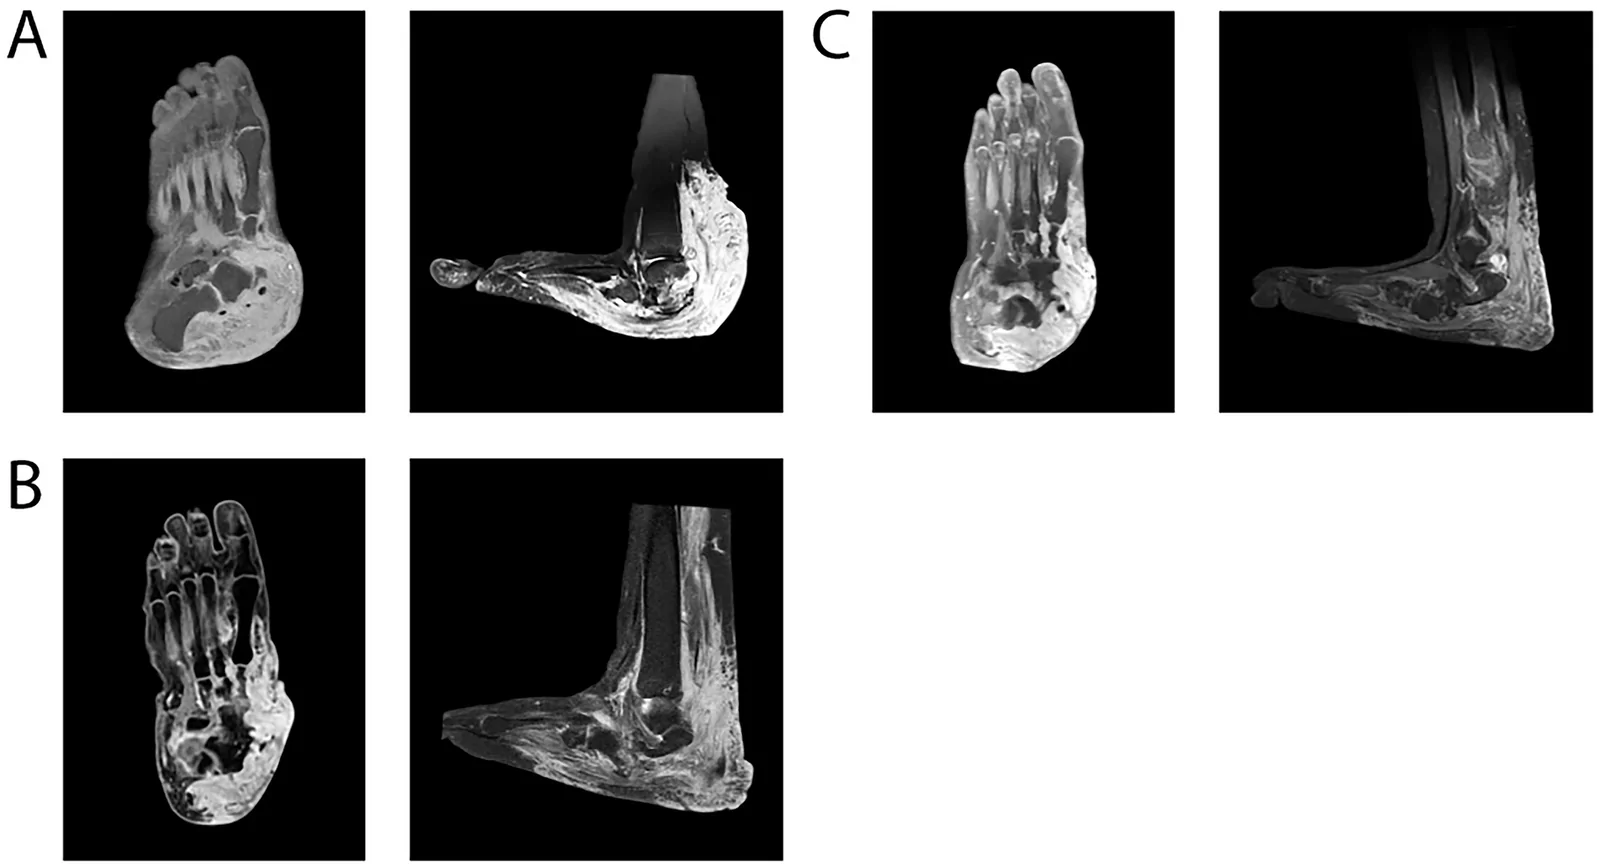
Magnetic resonance images in T1, T2, or diffusion mode after 9 months of selumetinib therapy (A), after 12 months of selumetinib therapy before surgery (B), and 40 months after selumetinib therapy and at 28 months post-surgery (C).
